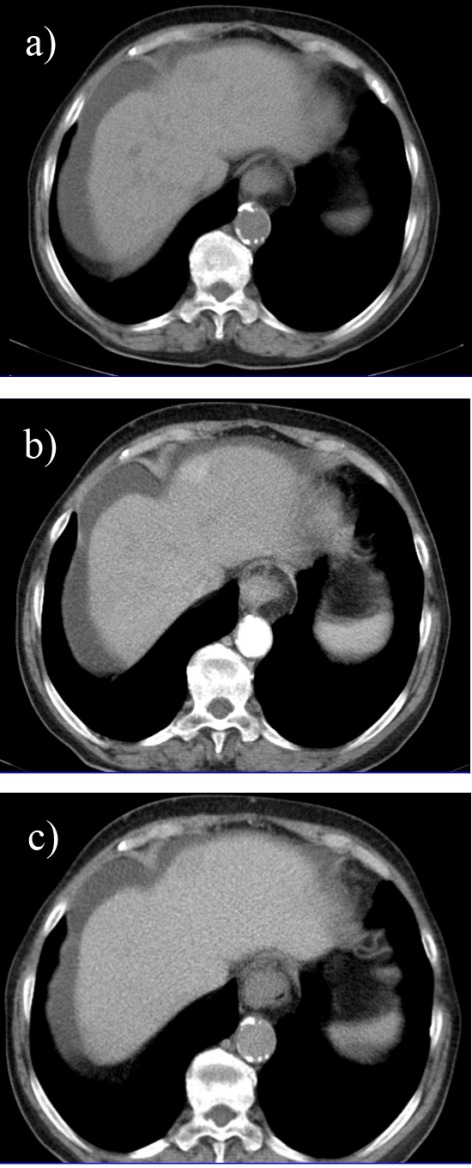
Triphasic multislice CT in a cirrhotic patient showing a moderatly differented small, subcapsular HCC in segment IV with slight hypoattenuation in the precontrast phase (a), with arterial enhancement (b) and washout in the portal phase (c);
